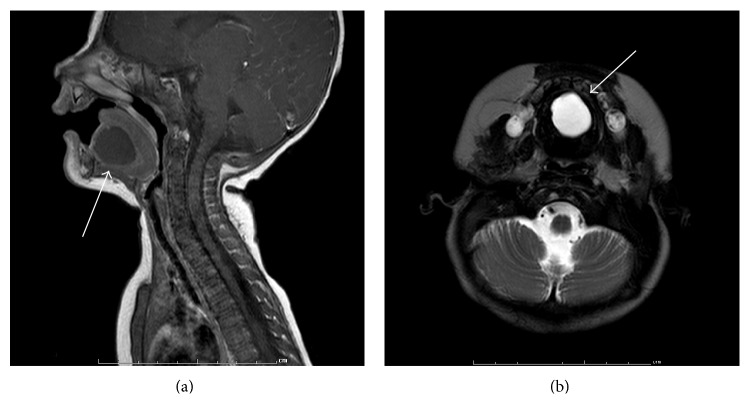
MRI. (a) T1-weighted, sagittal view: nonenhancing cystic mass in the sublingual space (arrow) without involvement of sublingual or submandibular glands. (b) T2-weighted, axial view: hyperintense mass midline in the anterior lower oral cavity (arrow).
